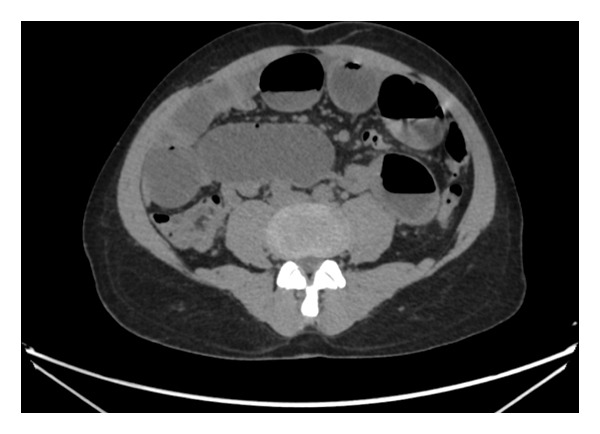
Unenhanced CT of the abdomen: axial images at the level of the mid‐abdomen demonstrating dilated fluid‐filled loops of small bowel.
